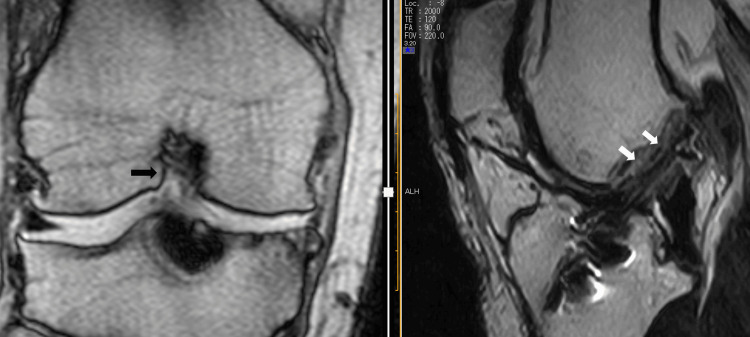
MRI showed narrowing of the lateral intercondylar notch (black arrow) and impingement of the graft (white arrows). MRI: magnetic resonance imaging
